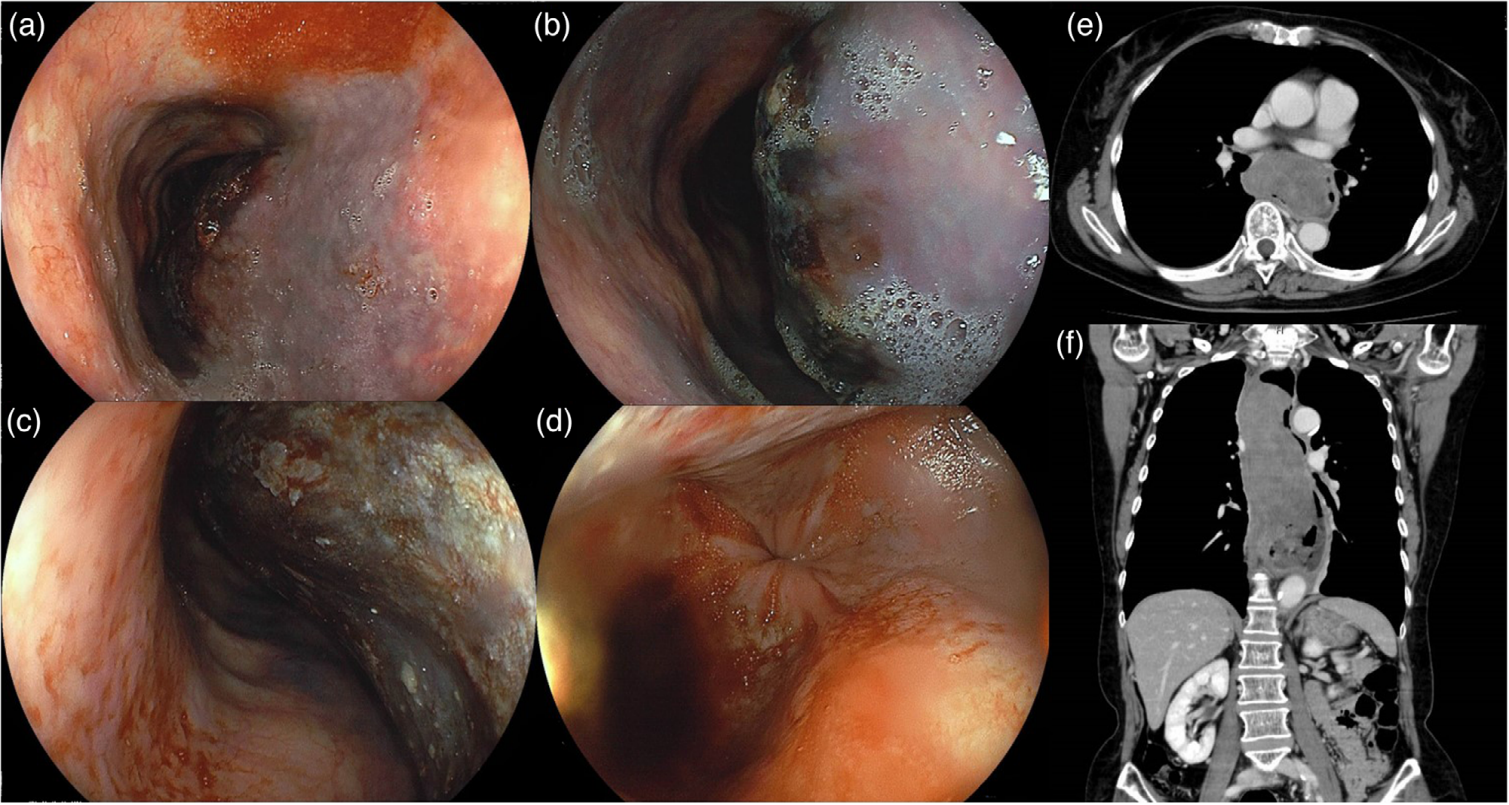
Esophagogastroduodenoscopy (EGD) showed a large intramural hematoma from upper esophagus to lower esophagus on the anterior wall (a‐c). Esophagogastric junction (EGJ) was tight and showed esophageal rosette sign (d). Chest and abdominal CT image of the esophageal intramural hematoma (EIH), which is a slight hyperdense mass in the dilated esophagus (e and f)
